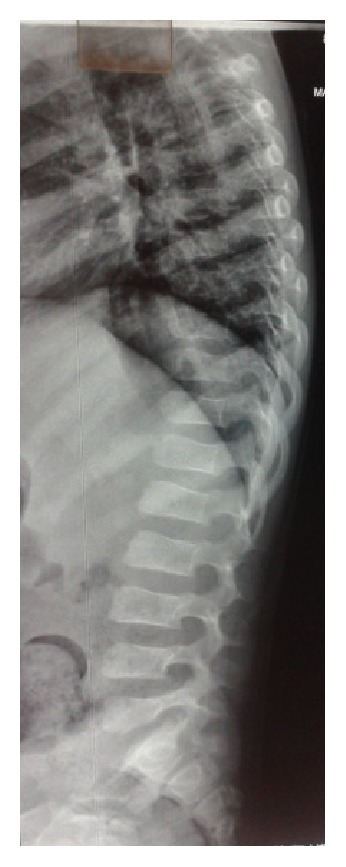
Lateral view of X-ray spine shows wedged shaped vertebral bodies with tongue-like anterior protrusion.
